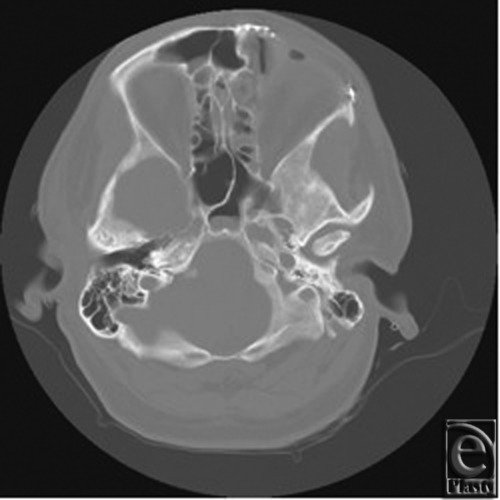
Postoperative computed tomographic scan showing supraorbital rim following reconstruction.
